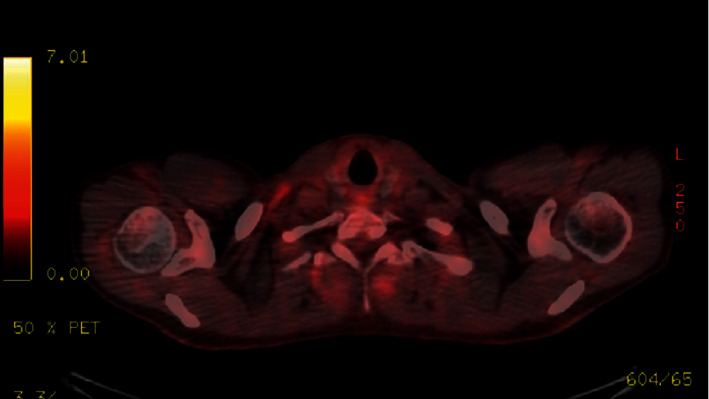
Immediate post treatment fused PET/CT. Seven weeks post initial head and neck CT. Approximate similar level demonstrating total resolution of the previous right heterogenous mass and right internal jugular compression. No fluorodeoxyglucose (FDG) avid lymph nodes seen.
